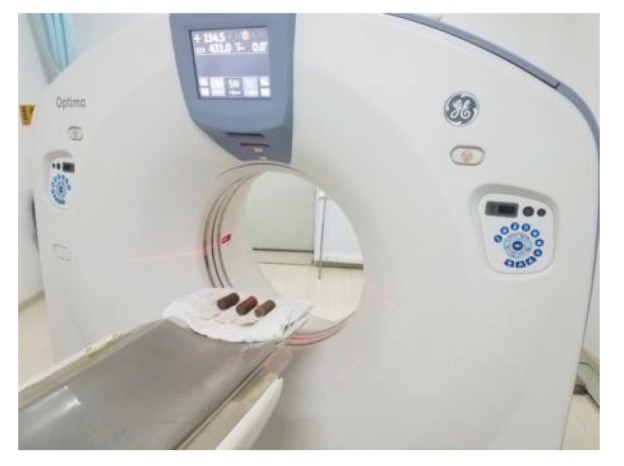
Type GE Optima 520 medical computed tomography (CT) scanner.
